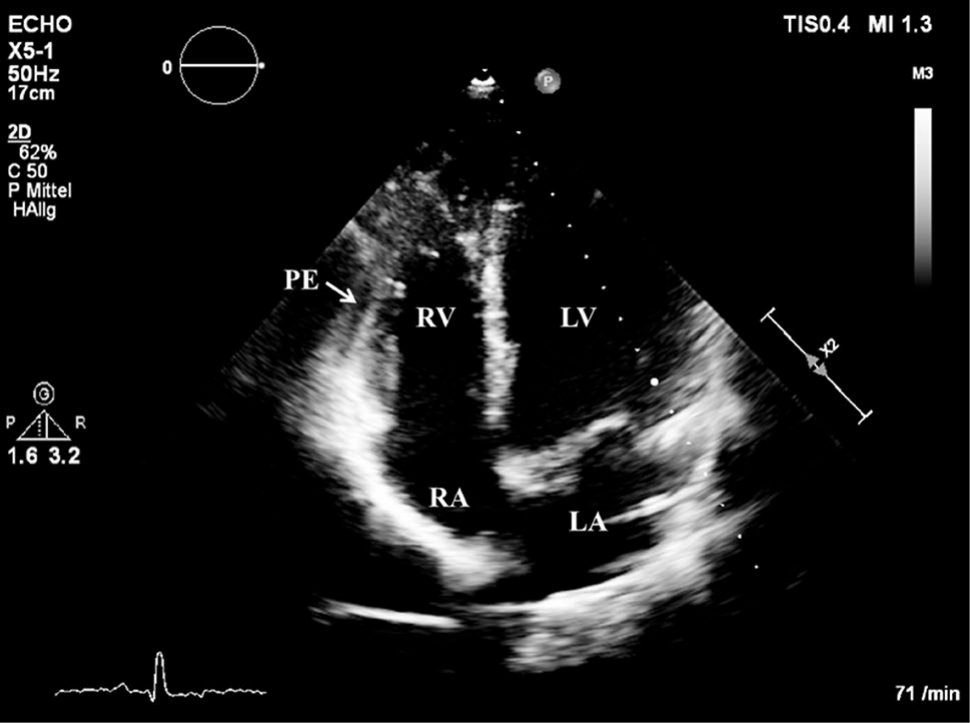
An echocardiography showed a pericardial effusion [PE]. Right ventricle [RV], left ventricle [LV], right atrium [RA], left atrium [LA]
